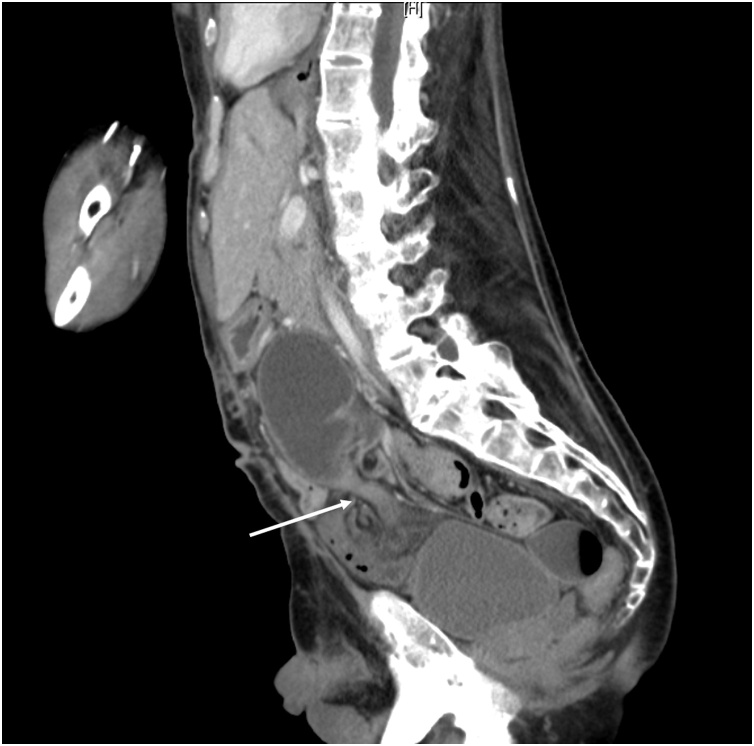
Sagittal section of abdominal CT image prior to the second surgery showed small bowel stenosis and no ossification in the previous surgical scar.
